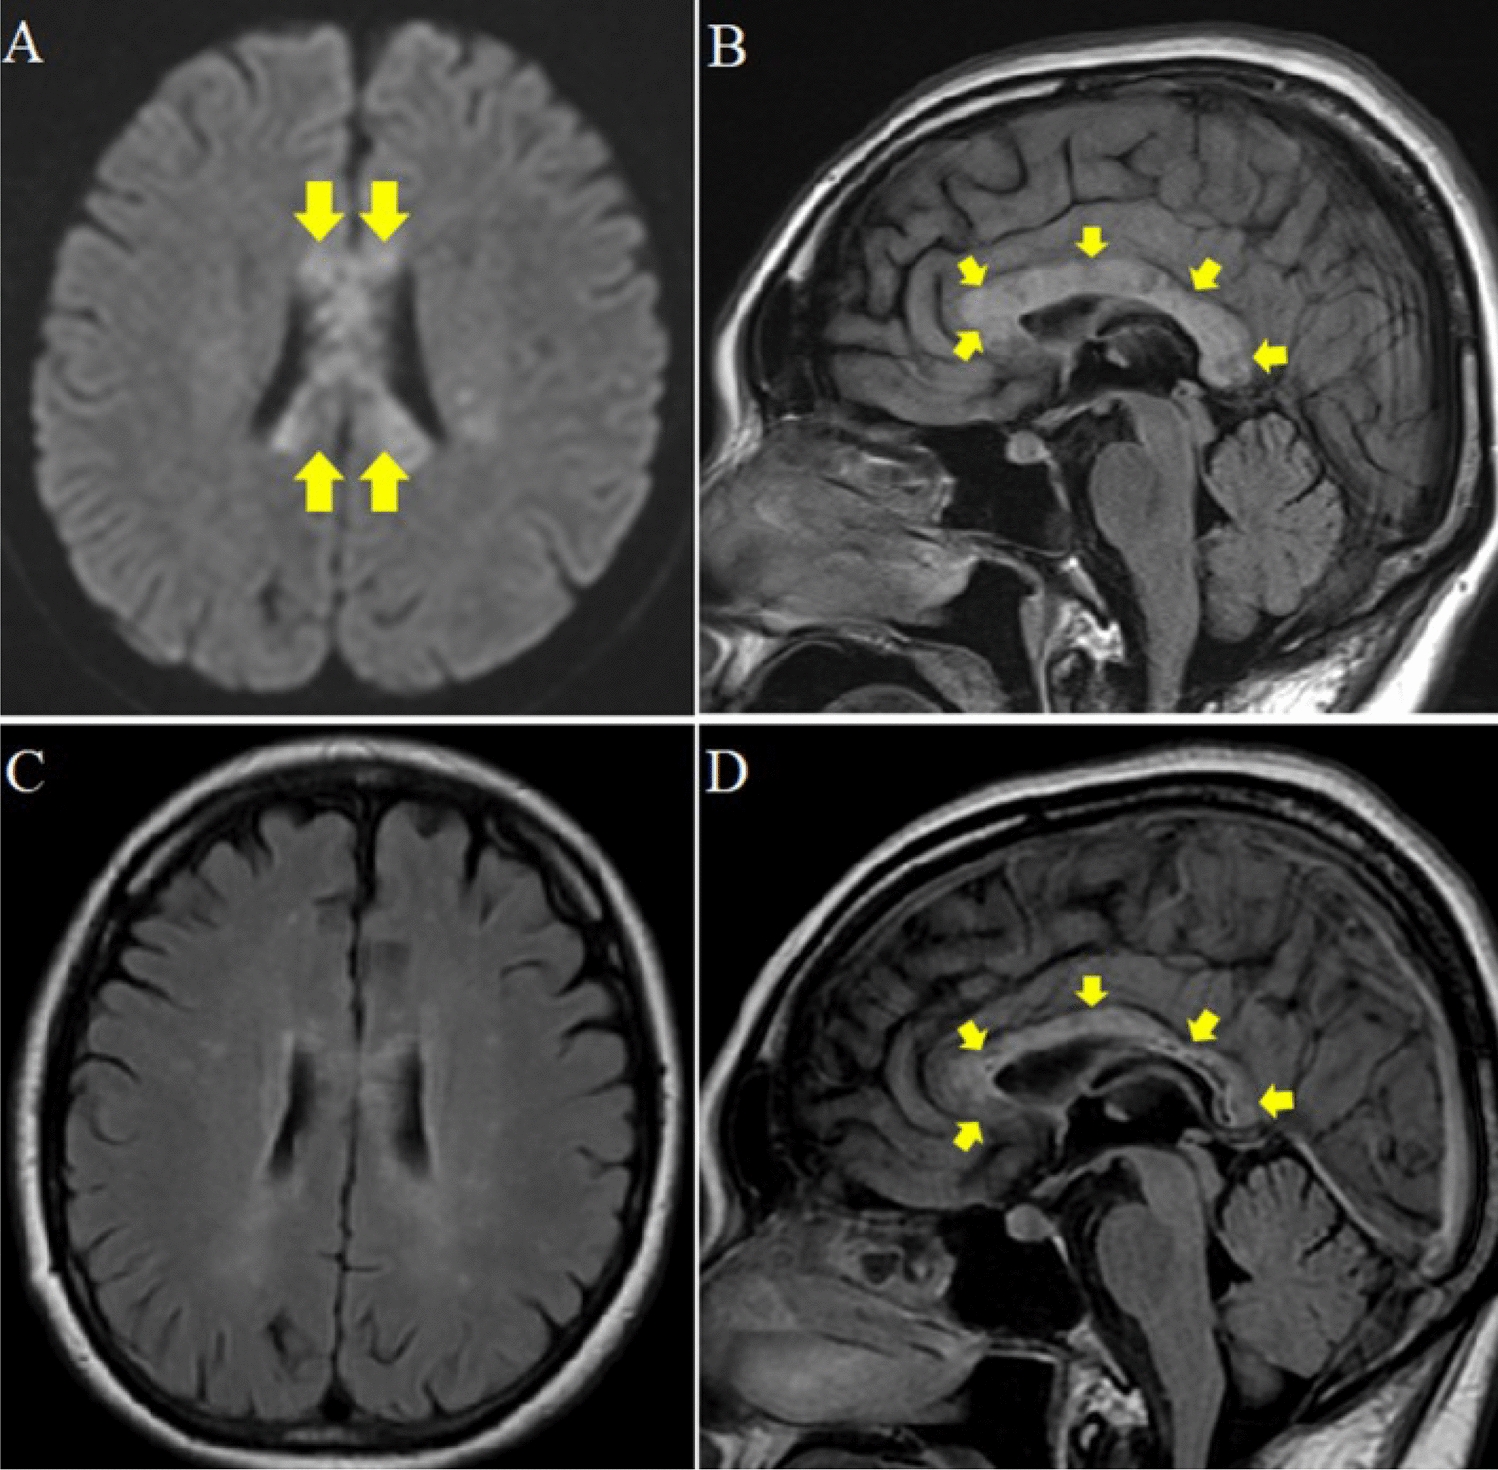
Splenial corpus-callosum lesions on DWI (patchy high signal (A)) & FLAIR (edema & regression (B)), arrow indicates irregular signals and low intensity at the core; FLAIR: Fluid Attenuated Inversion Recovery, DWI: Diffusion-weighted imaging. [20] Copyright © 2018 Shozawa et al.
